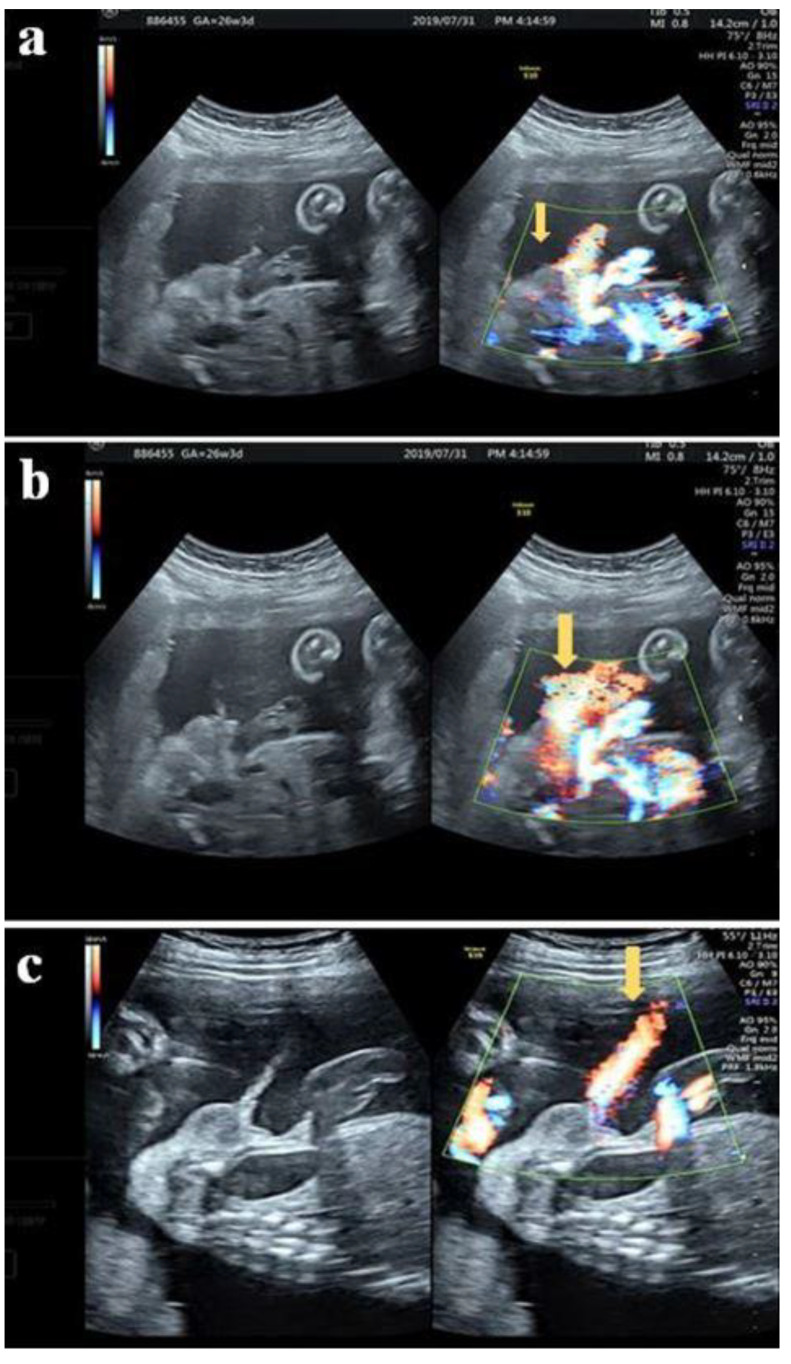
(a) The same patient at 26 + 3 weeks with stream of “coarse” urinary jets seen initially under color Doppler (arrow) (b) Typical fanning stream of the urinary jet revealed follows the initiated “coarse” jet of urinary stream (arrow) (c) Normal voiding under color Doppler with “linear” jet of stream (arrow).
